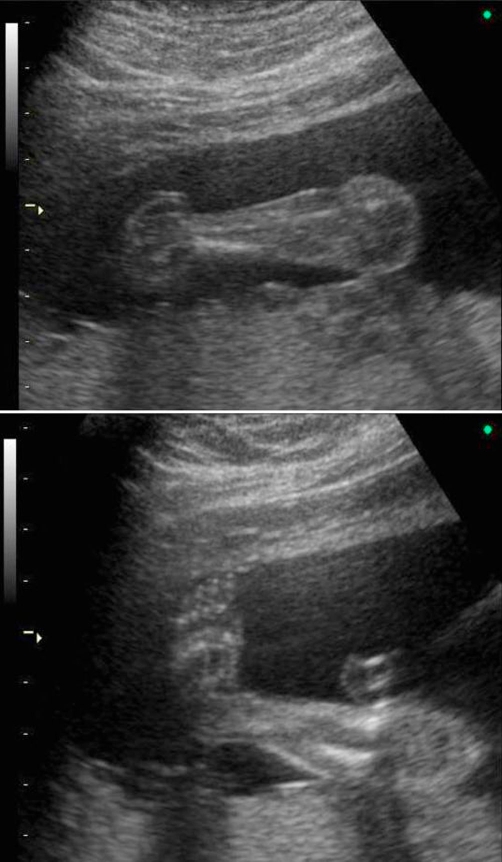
Ultrasound scan showing talipes equinovarus.
